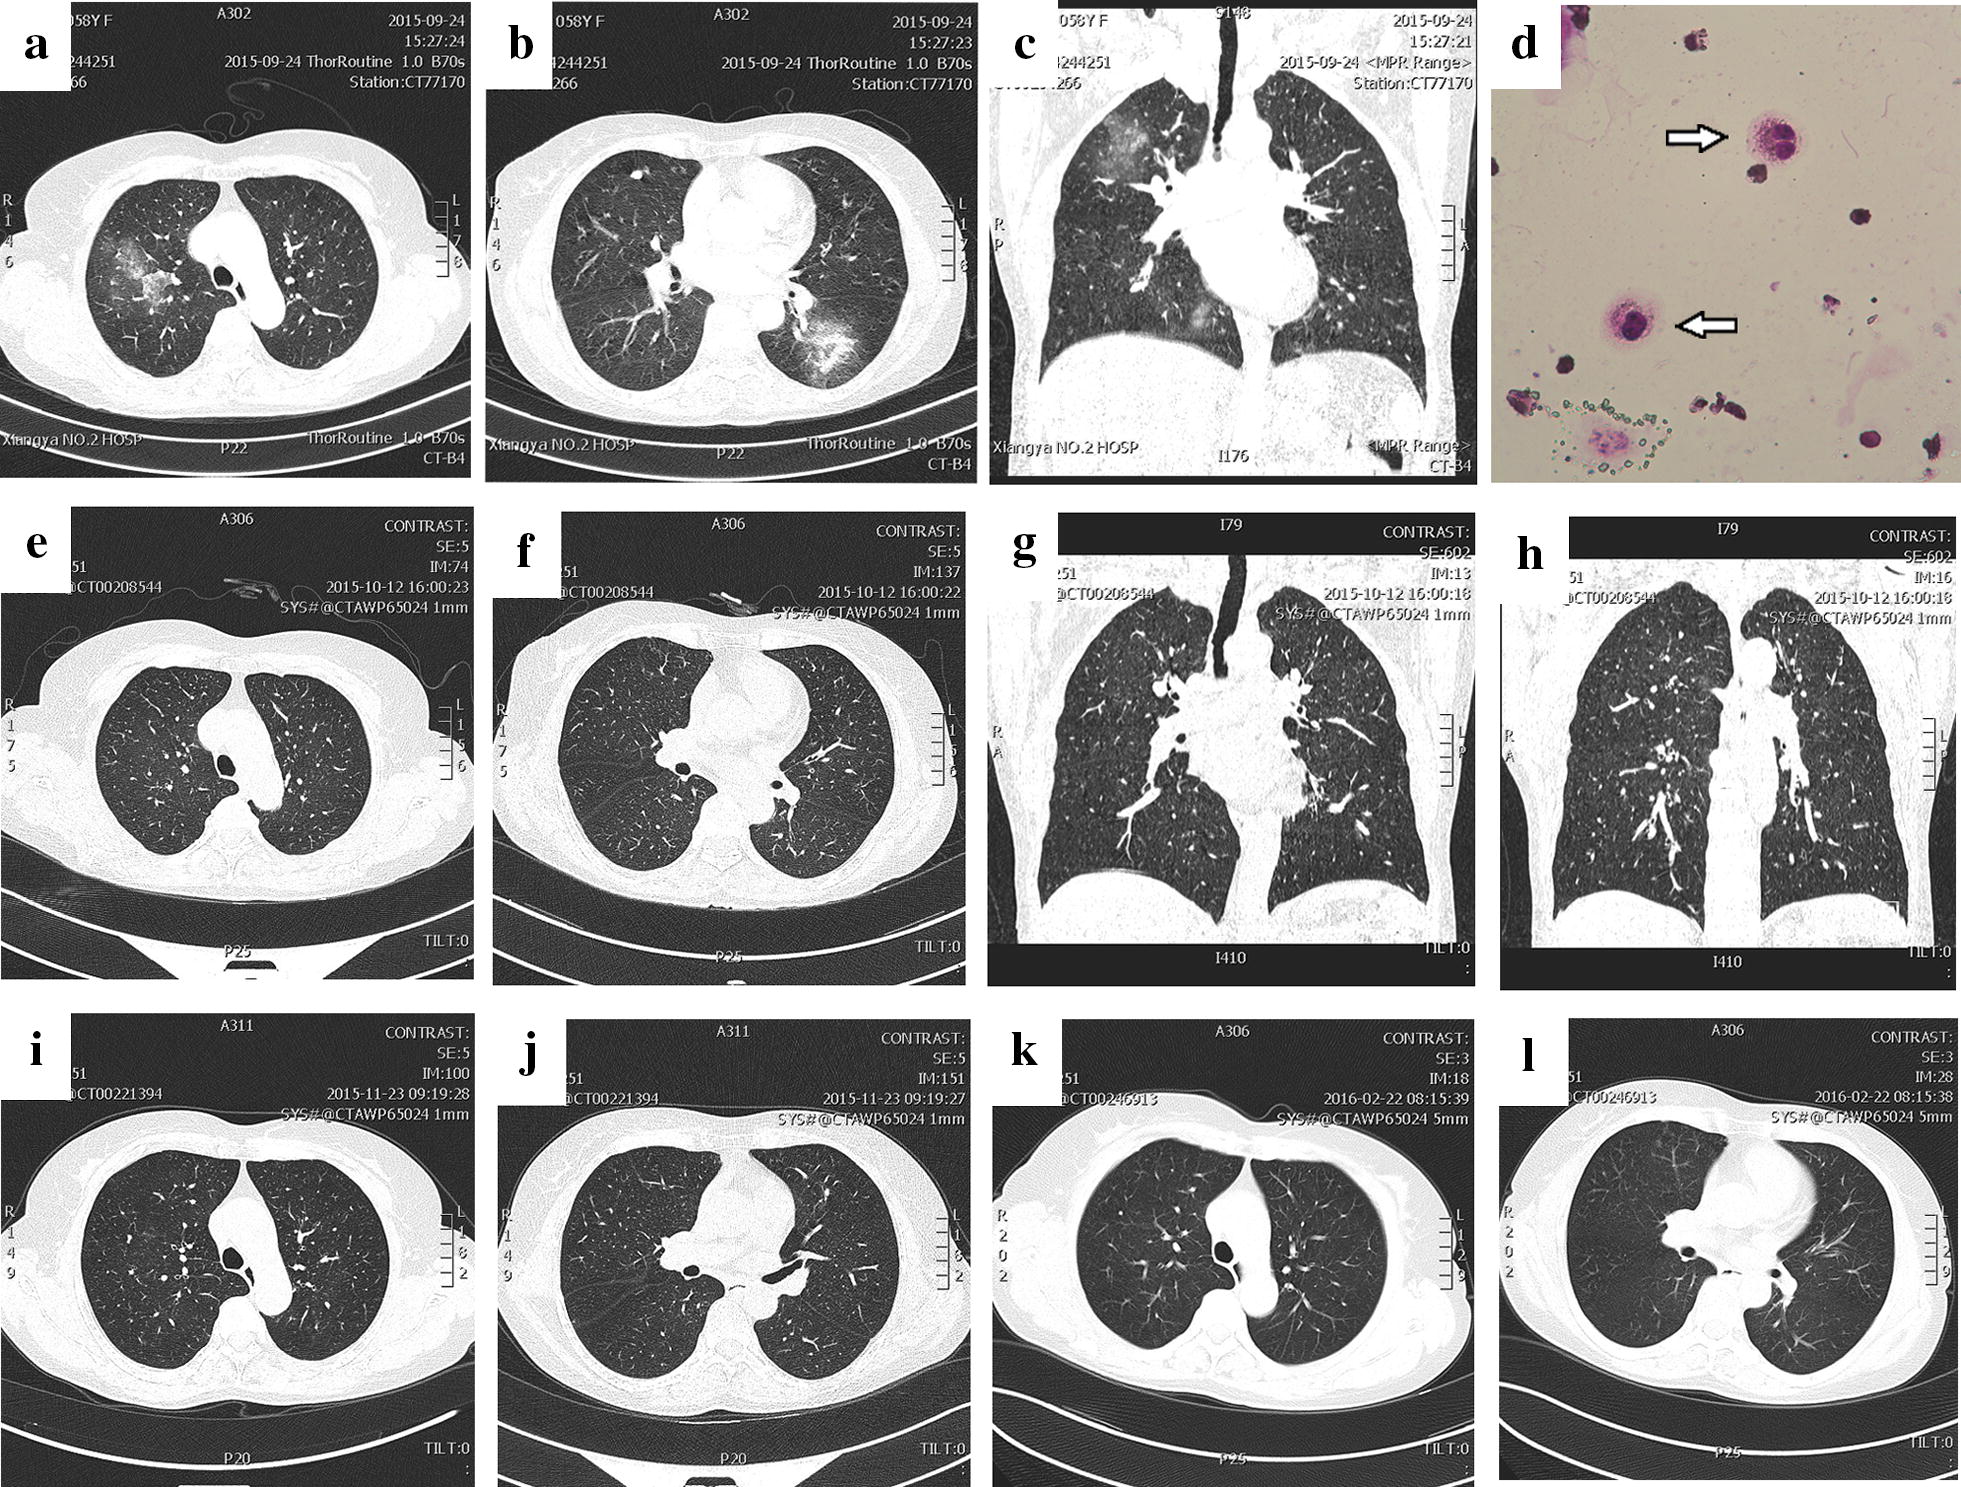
Chest CT images and eosinophils in BAL. a–c CT scans showed multiple infiltrates in the lower left lung and ground-glass opacities in the subpleural regions of both upper lung fields; d eosinophils were observed in BAL (arrow); e–h double-lung disease was largely absorbed after 2 weeks; i, j pulmonary CT scan indicated improvement and resolution of the lesions 2 months later; k, l CT scan after 5 months
